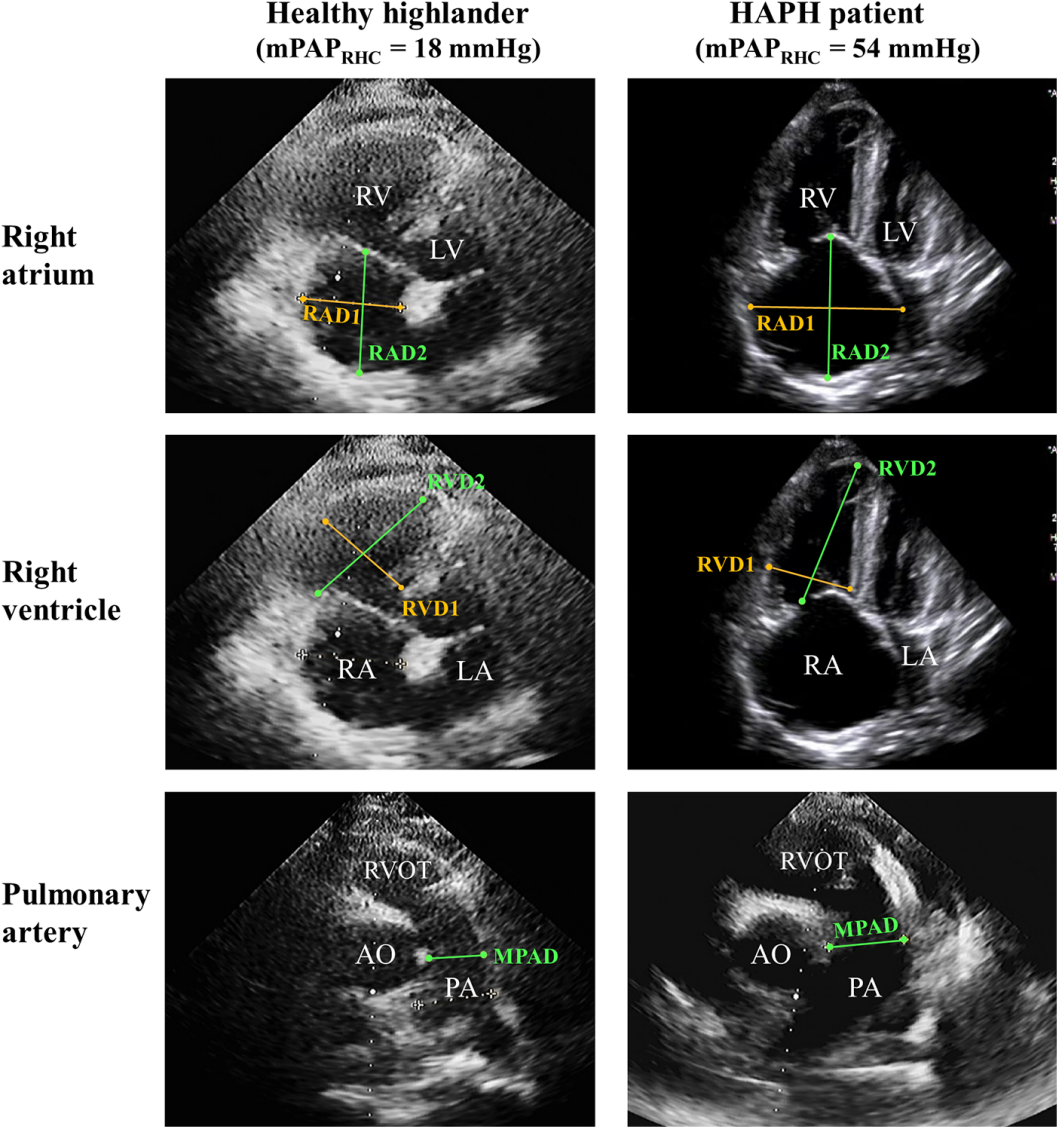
Representative imaging of the echocardiographic sign of right heart dysfunction. Right ventricle-focused apical four-chamber view and pulmonic valve-focused parasternal short-axis view of HH and HAPH patients. PA, pulmonary artery; RAD1, transverse diameter of right atrium; RAD2, vertical diameter of right atrium; RVD1, transverse diameter of right ventricle; RVD2, vertical diameter of right ventricle.
